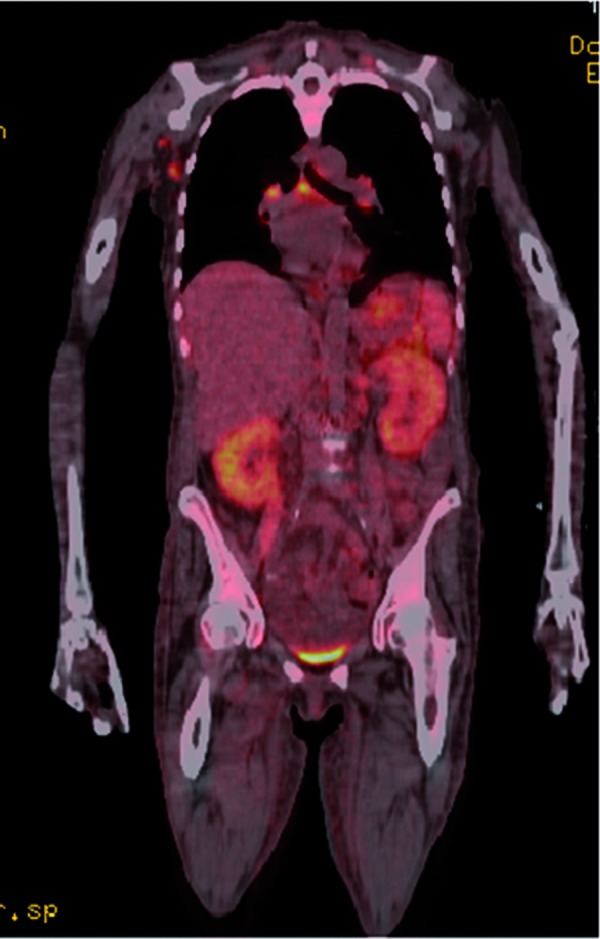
Positron emission tomography (FDG-PET) scan in a patient with acute interstitial nephritis (AIN) in the setting of drug rash with eosinophilia and systemic symptoms (DRESS) syndrome.
